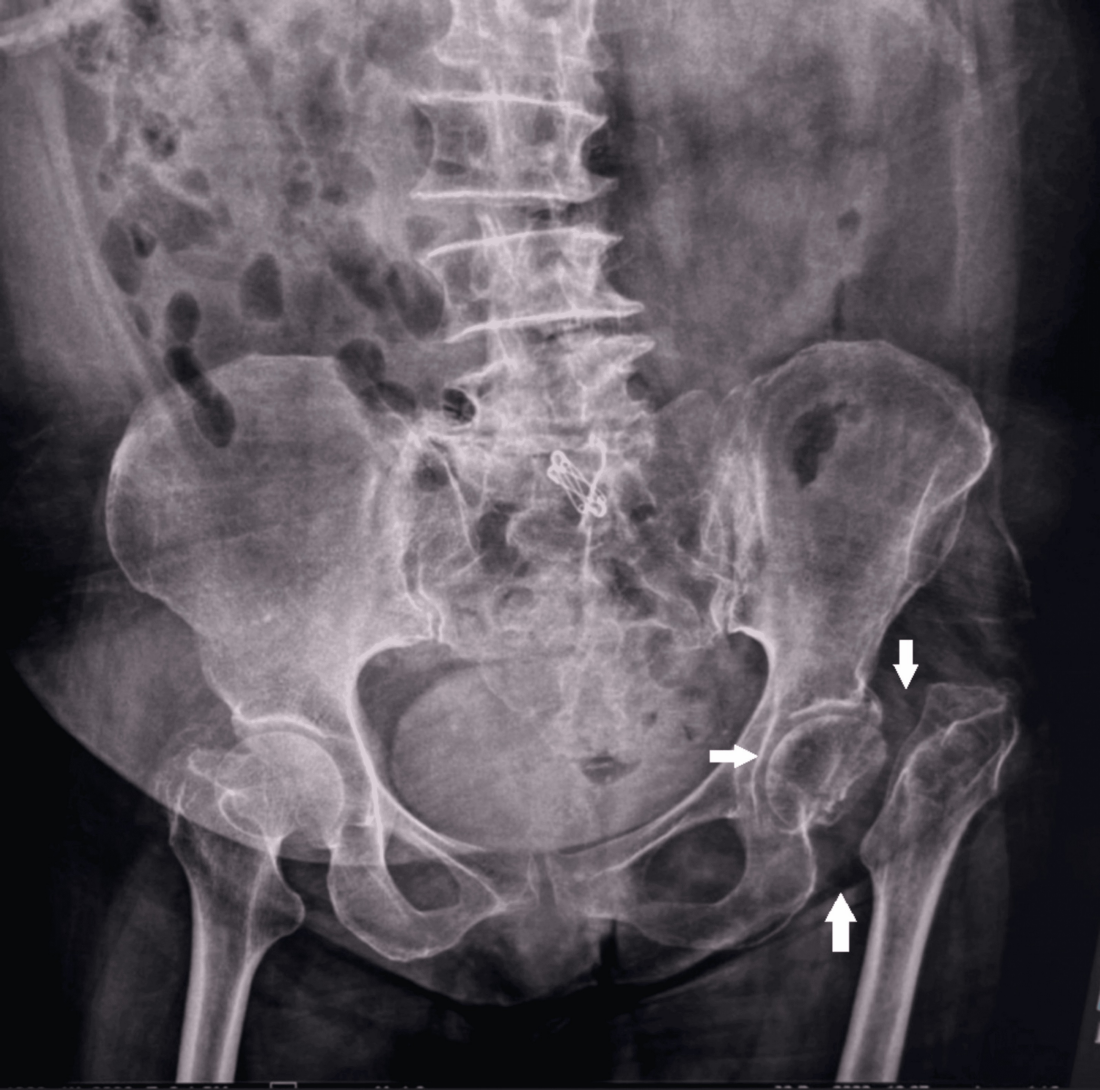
A standard frontal X-ray of the pelvis showing the pathological fracture of the left femoral neck
